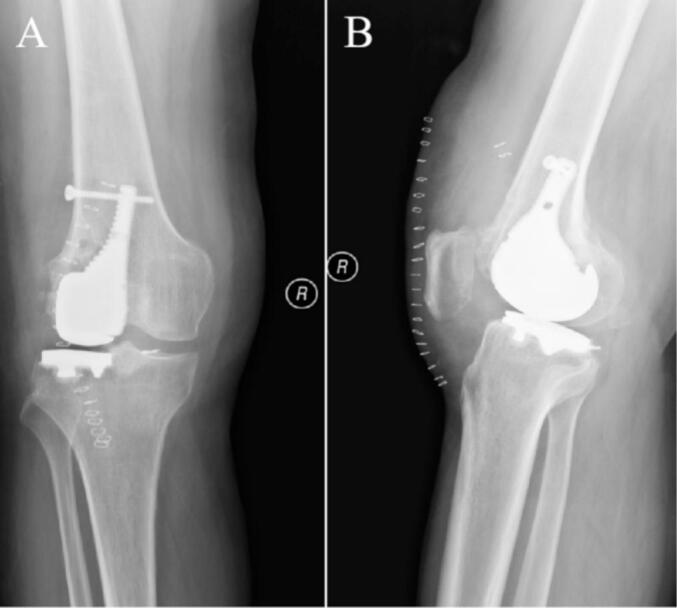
The pre-discharge X-ray images. (A) AP view of the right knee. (B) Lateral view of the right knee.
